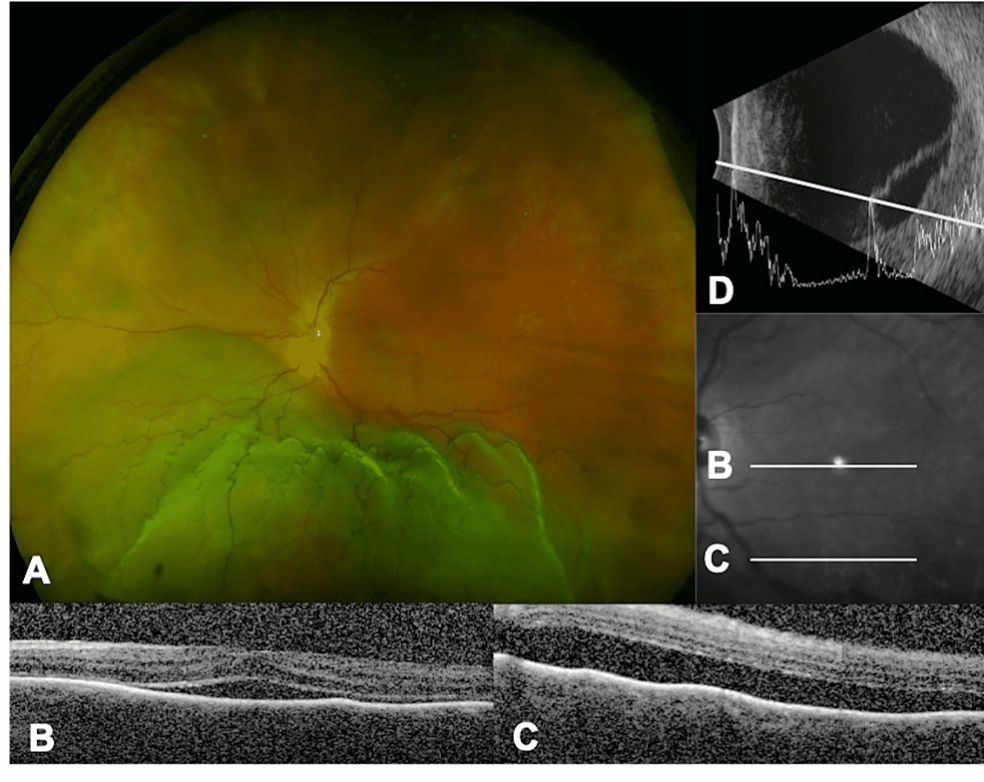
Fundus photo, Spectral domain-OCT and B-scan of left eye Examination at presentation showed an inferior retinal detachment of the left eye with no clear retinal breaks or masses (A). Spectral domain-OCT revealed an inferior retinal detachment of the left eye with a thickened choroid and sub-retinal fluid involving the fovea (B) and extending inferiorly with the lump bumpy appearance (C). B-scan ultrasonography confirmed a diffuse inferior choroidal mass measuring 5 mm at the apex with overlying retinal detachment (D). OCT: optical coherence tomography
